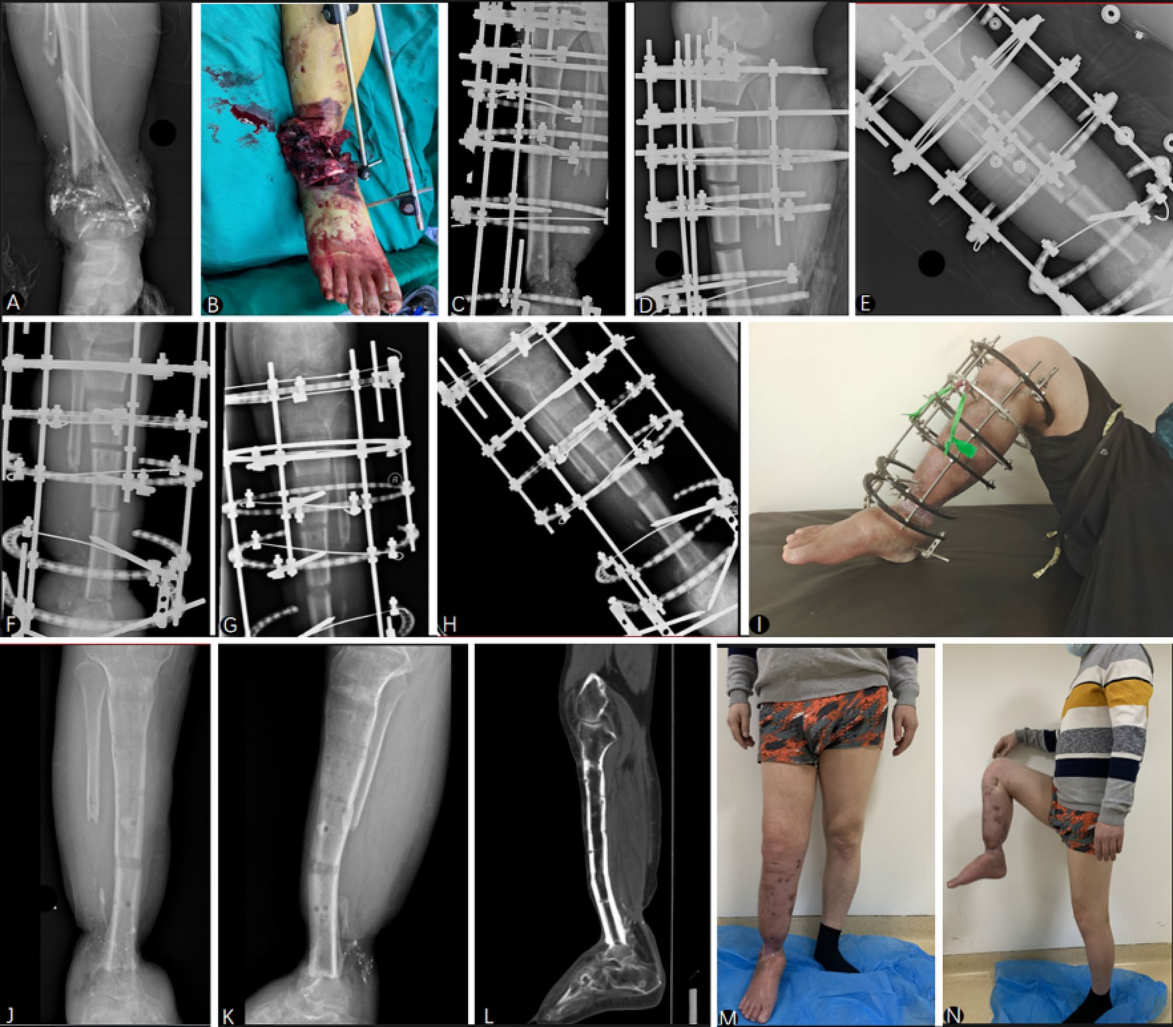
A Initial anteroposterior radiograph of a 38-year-old male outlining the preoperative condition. B Intraoperative image showcasing the severity of tissue damage. C Immediate postoperative anteroposterior radiographs showing the initial phase of external fixation. D, E,F, G,H Progressive radiographic sequence highlighting the stages of pentafocal bone transport, showcasing the initial external fixation, the progressive bone transport, and the stages of bone consolidation. I Clinical photograph of the external fixator in place, demonstrating the patient’s limb alignment during treatment. J, K,L Anteroposterior and lateral radiographs after the completion of the bone transport, indicating the new bone growth and alignment. M, N Final clinical photographs of the patient demonstrating the range of motion and improved functional capacity of the lower limb at the last follow-up
